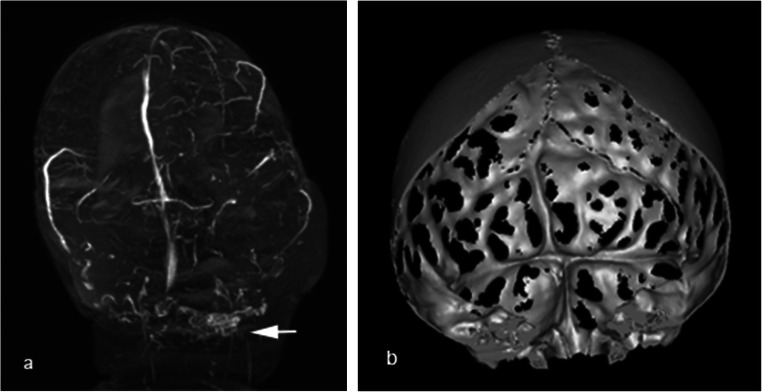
Anomalous anatomy in patients with craniosynostoses: a abnormal venous anatomy according to MR angiography images. B Appearance of the inner surface of the calvaria in a patient with Crouzon syndrome, 3D reconstruction of the preoperative CT scan
